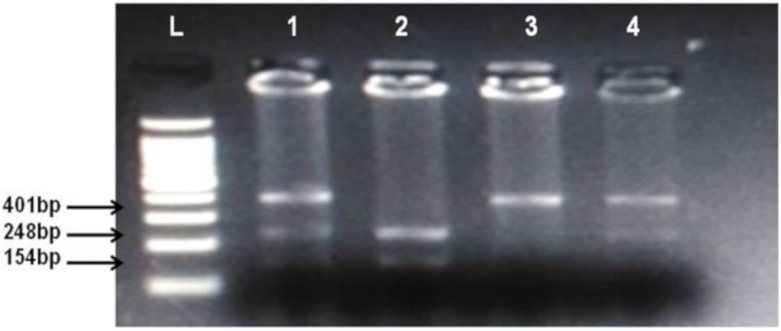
Identification of cannabinoid receptor 1 (CNR1) rs806368 polymorphism. Lanes L, DNA ladder, 1 and 4 show CT heterozygotes (Cleaved PCR product 248 bp, 154 bp and uncleaved PCR product 401 bp), 2 CC homozygote (cleaved PCR product into 248 bp and 154 bp fragments), 3 TT homozygote (uncleaved PCR product 401 bp).
